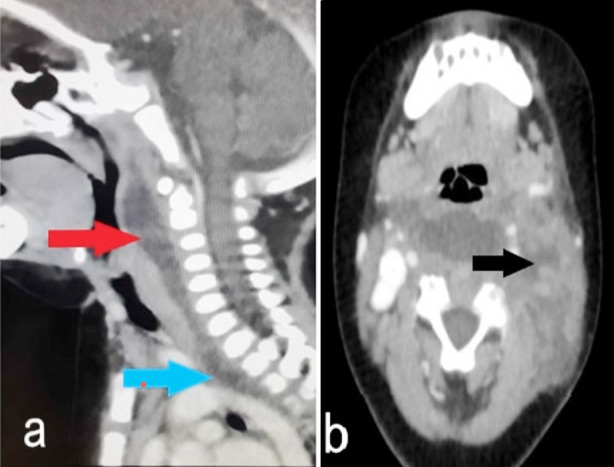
CT scan of the neck with contrast. (a) Multi loculated large retropharyngeal access collection seen extended into the parapharyngeal space more toward left side involving left posterior triangle (Red arrow). The abscess extending into the anterior and posterior mediastinum (Blue arrow). (b) Non-visualised left deep jugular vein that indicate presence of thrombus (black arrow).
